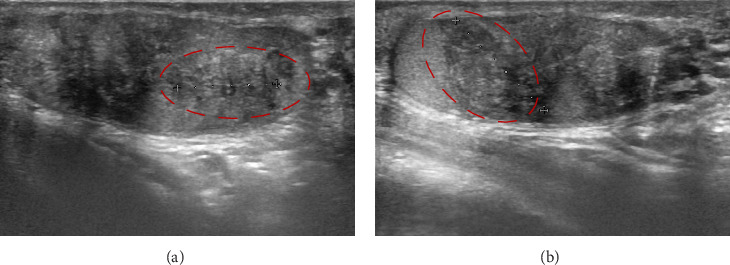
The testicular ultrasound of the right testis showing bilateral TARTs appearing as pseudonodular areas of rounded morphology and ill-defined margins affecting the entire parenchyma of respectively of (A) 17 mm and (B) 12 mm with a predominantly inhomogeneous hypoechoic echostructure with hyperechoic spots. These lesions appeared highly vascularized and are consistent with TARTs, despite sometimes they could be placed into differential diagnosis with other tumor lesions.
